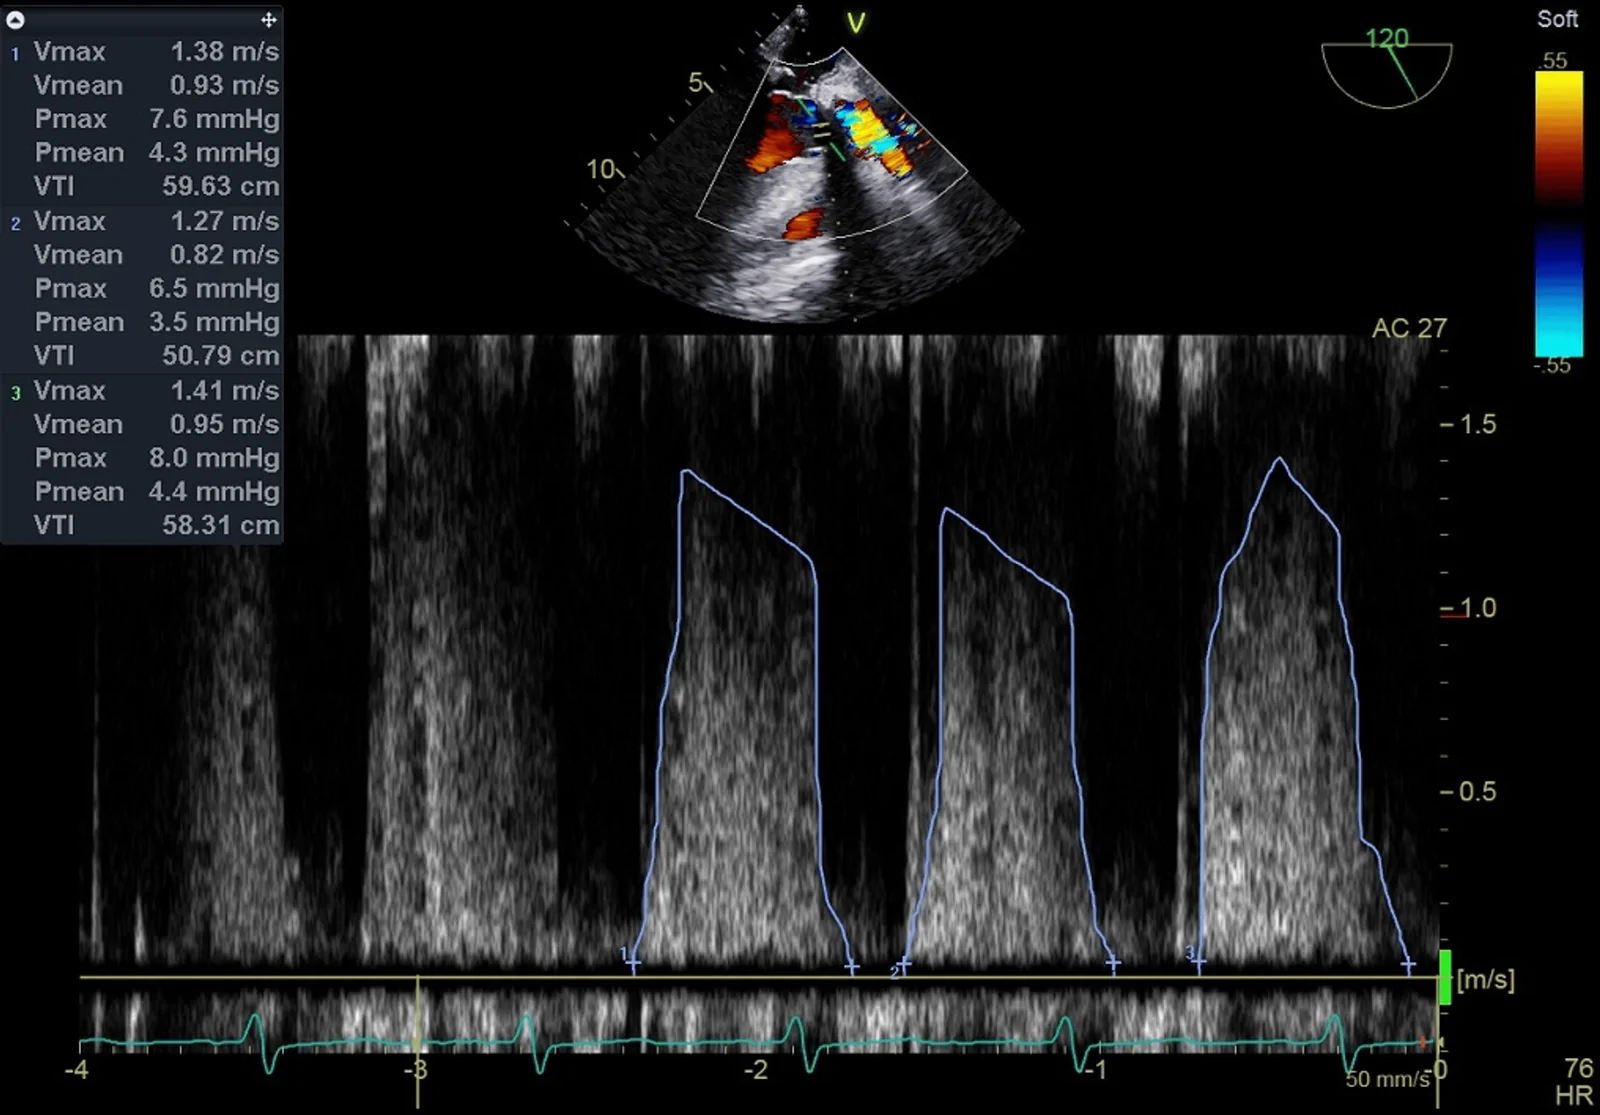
Transesophageal echocardiogram showing a long-axis view of the aortic valve after sternal closure. We used color Doppler imaging to trace the physiological regurgitation flowing from a mechanical prosthetic valve implanted at the aortic valve site into the left ventricle during diastole. By tracing the three physiological regurgitation waves, we measured the average pressure difference, which was found to be 4.3, 3.5, and 4.4 mmHg; we calculated the average value to be 4.07 mmHg.
